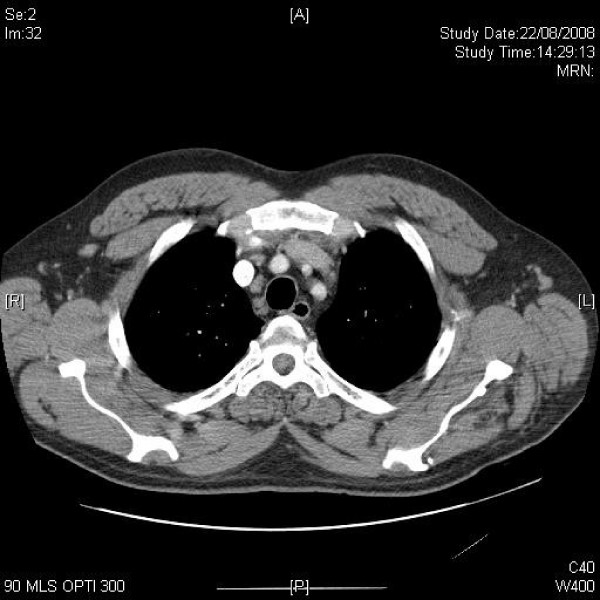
Axial CT of upper chest shows mildly enlarged axillary and mediastinal lymph nodes.
